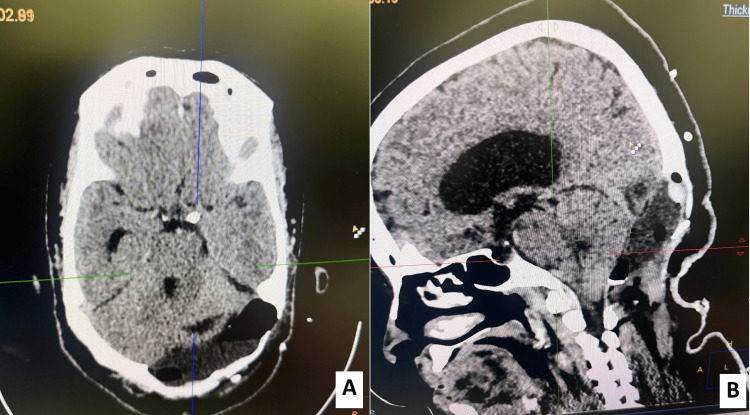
Postoperative CT scan. A: Axial non-contrast CT image showing postoperative adequate decompression of the posterior fossa and no residual mass effect, with near-complete resection of the epidermoid cyst. B: Sagittal CT reconstruction demonstrating re-expansion of the fourth ventricle and absence of acute hemorrhage or obstructive hydrocephalus, consistent with a favorable postoperative outcome.
